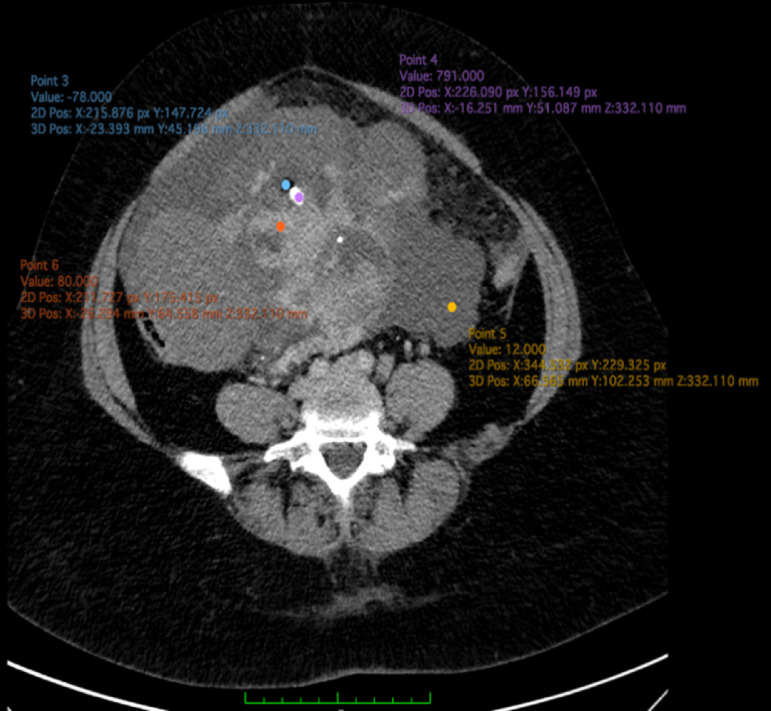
Axial CT view in venous phase, revealing a dominant cystic mass (12 HU, yellow dot) located on the right ovary, with papillary projections, a solid component (80 HU, orange dot), calcification (791 HU, purple dot), and a fat component (-78 HU, blue dot). The mass is multilobulated, with a smooth margin measuring approximately 10.0 × 15.6 × 22.6 cm. Ascites can also be observed in the abdominal and pelvic cavity (asterisk) (Color version of the figure is available online.)
